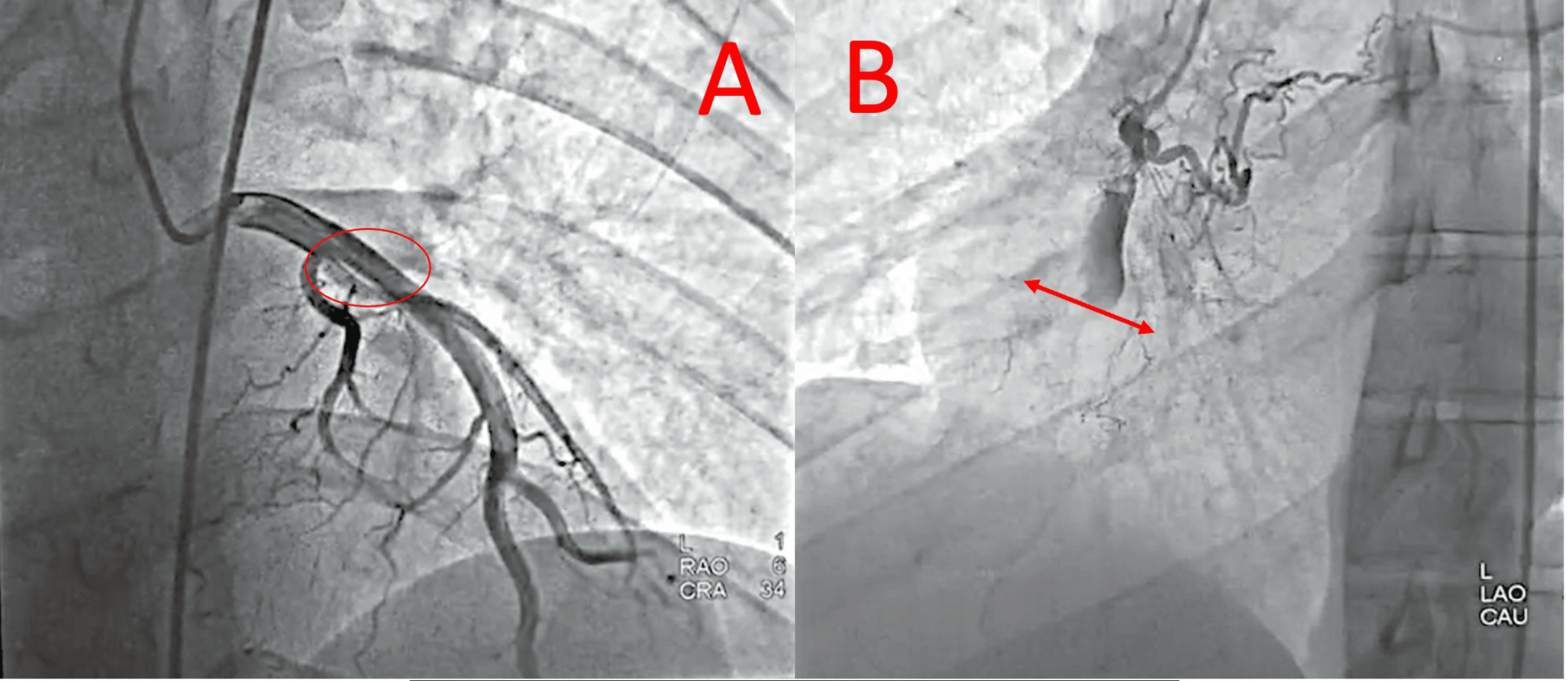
Coronary angiography: (A) Mid-LAD plaque (red circle), and (B) the chronic right coronary artery (RCA) occlusion (the red arrow), correlating with the ischemic MRI findings. Images were obtained from the authors’ institutional database. LAD, left anterior descending; RCA, right coronary artery; MRI, magnetic resonance imaging
